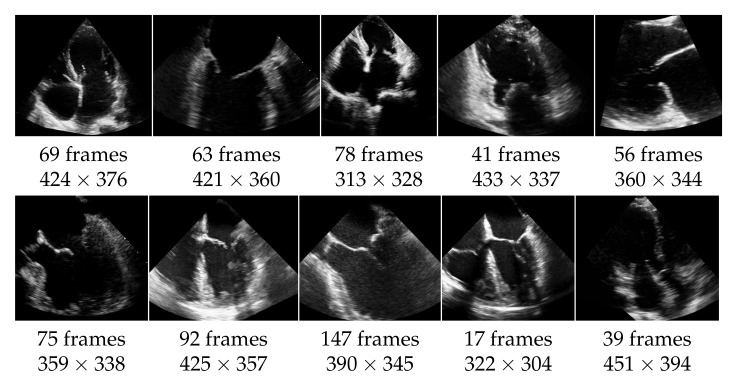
Dataset of 10 echocardiographic videos, with their number of frames and their spacial resolution.
